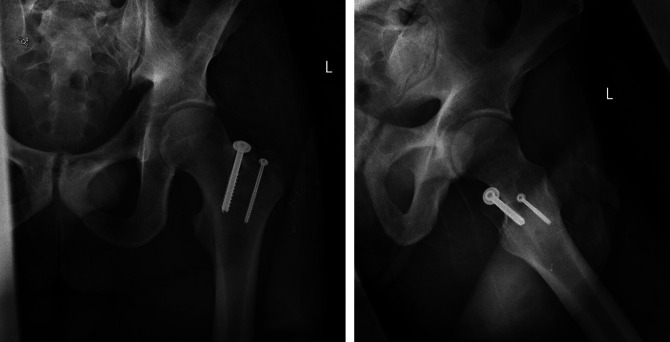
AP and lateral radiographs of the left hip demonstrating anatomic reduction of the left greater trochanteric fracture with stable parallel implant.
